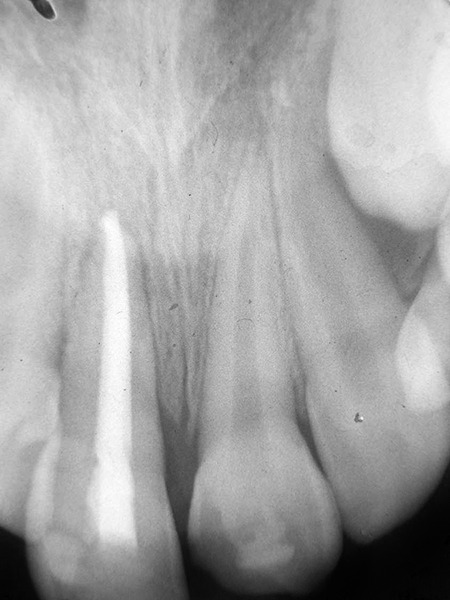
Intraoral radiograph―40 months after replantation
